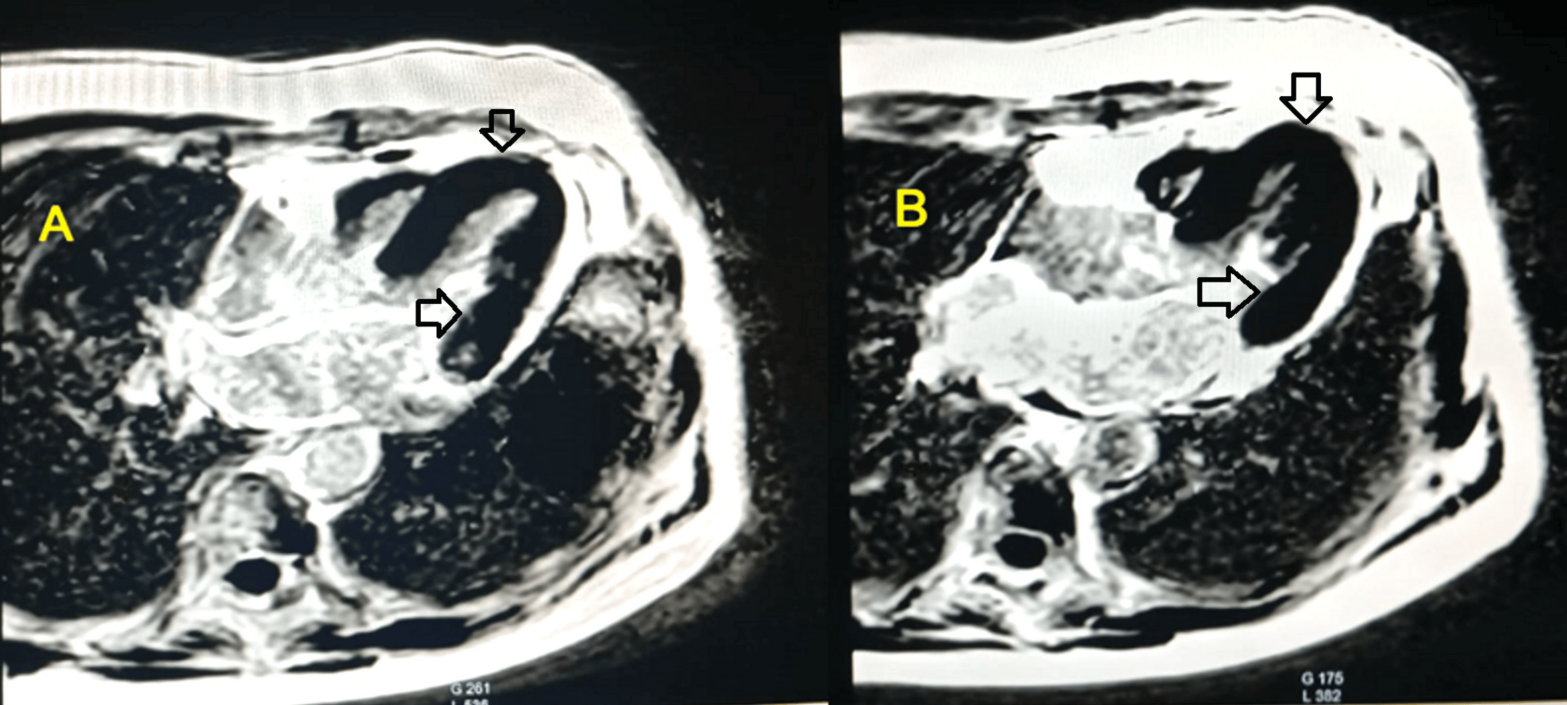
Axial MRI images showing the absence of late enhancement in the myocardial wall after gadolinium injection, in diastole (A) and systole (B), with arrows pointing to the left ventricular myocardial wall
